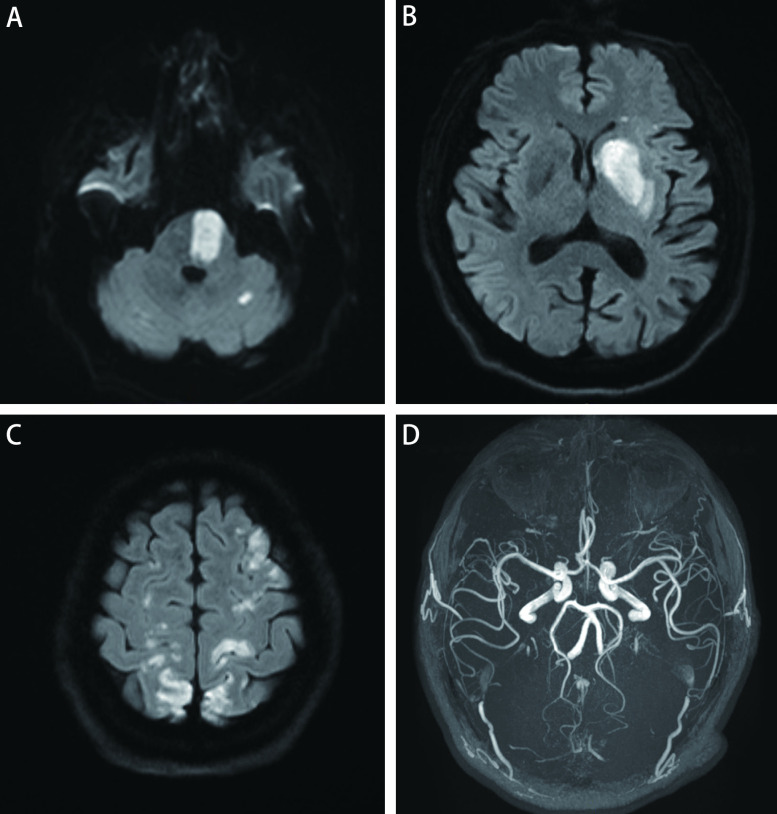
病例9的DWI及MRA图像特征。A-C：双侧额叶、顶叶、左侧基底节区、脑桥及左侧小脑半球可见多发高信号，提示梗死；D：颅内动脉管腔未见明显狭窄。 DWI and MRA feature of case 9. A-C: DWI shows multiple foci of high signal intensity in bilateral frontal lobe, parietal lobe, left basal ganglia, pons and left cerebellar hemisphere, indicating infarction; D: MRA shows no significant narrowing of all the intracranial arterial lumen. DWI: diffusion-weighted imaging; MRA: magnetic resonance angiography.
